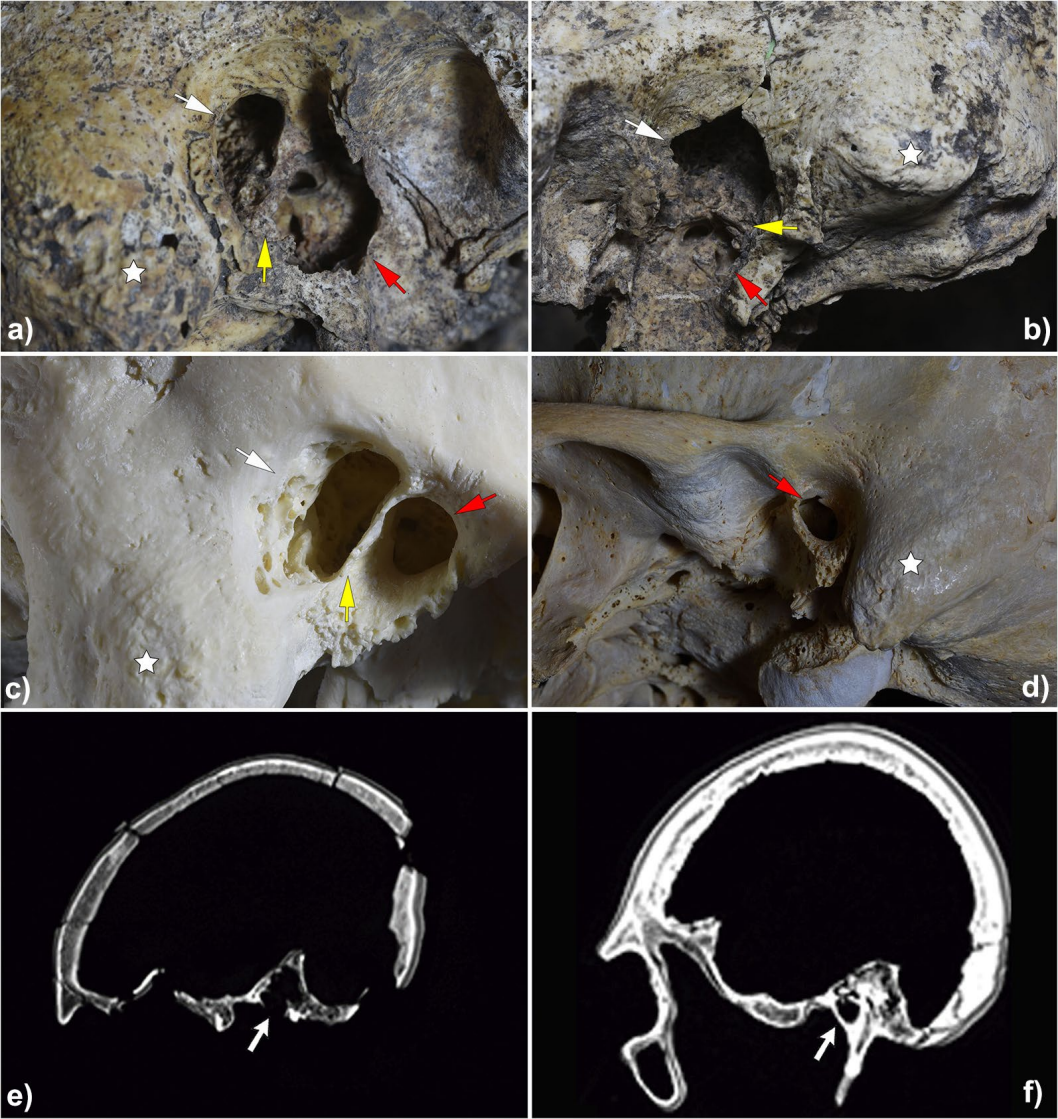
Computed tomography scans and details of both temporal bones of the skull under study and some samples of the comparative analysis. Superior: Details of external auditory region on the right (a) and the left (b) temporal bones of the skull under study. It is to be noted the deterioration of the tympanic cavity in both temporal bones due to taphonomic processes. Middle: Present-day skull with mastoidectomy performed by the students of the Faculty of Medicine of the University of Valladolid (c) and example of an archaeological skull without any pathology used for comparative analysis (d). Red arrows indicate the external auditory canal. White arrows point to bone erosion in the postero-superior part of the external auditory canal due to the mastoidectomy. Yellow arrows indicate the *scutum*—thin bony spur that is formed by the superior wall of the external auditory canal and the lateral wall of the tympanic cavity. *Mastoid process. Inferior: Computed tomography (CT) scans of para-sagittal sections at right middle ear level—the arrows point to the middle ear—from the skull under study (e) and from a present-day skull without pathology (f).
